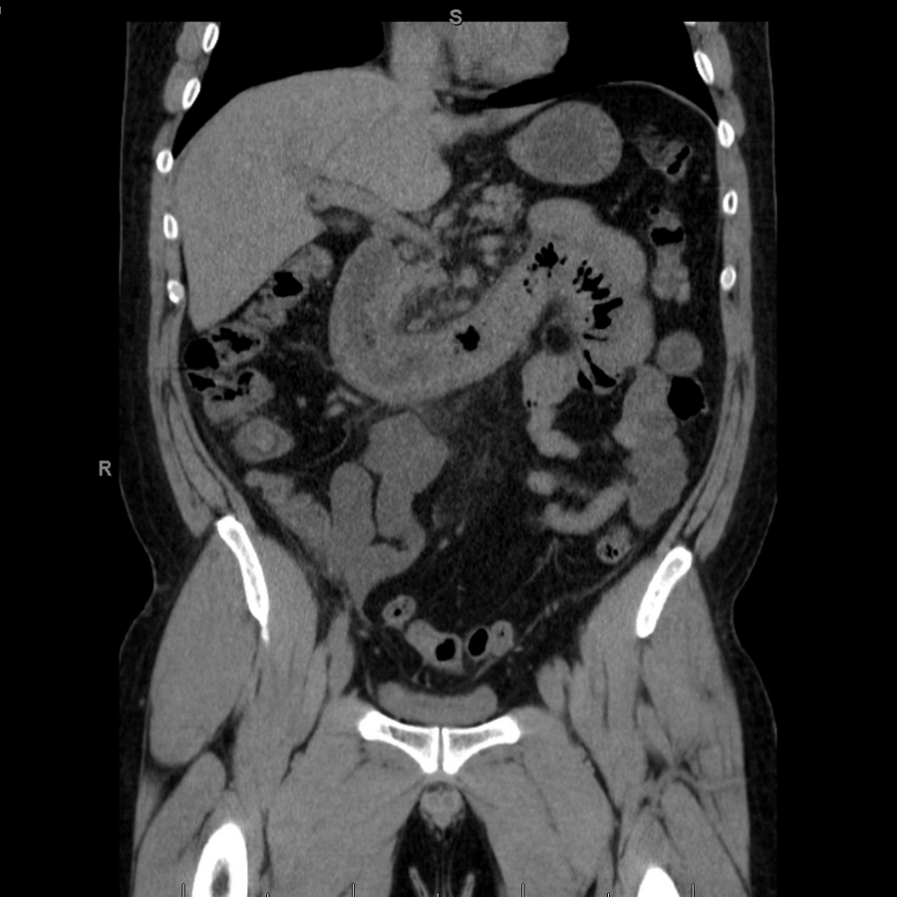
Coronal section of CT abdomen and pelvis with contrast showing bowel wall inflammation and edema.
